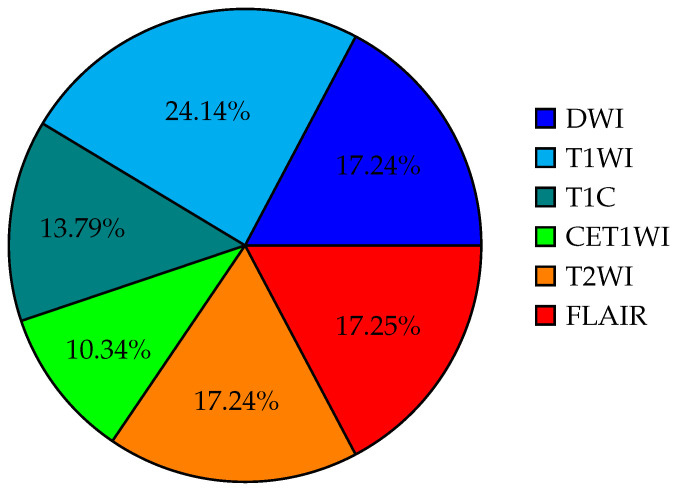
Percentage distribution of the use of different types of MRI signals in the selected studies.
